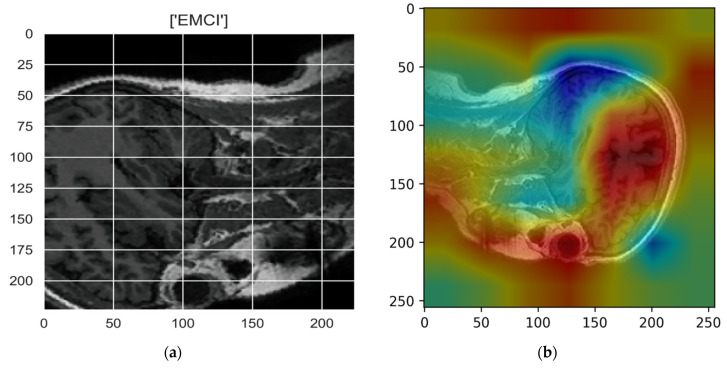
Grad-CAM explanation for the prediction of class EMCI: (a) MRI image, (b) Grad-CAM attention map.
